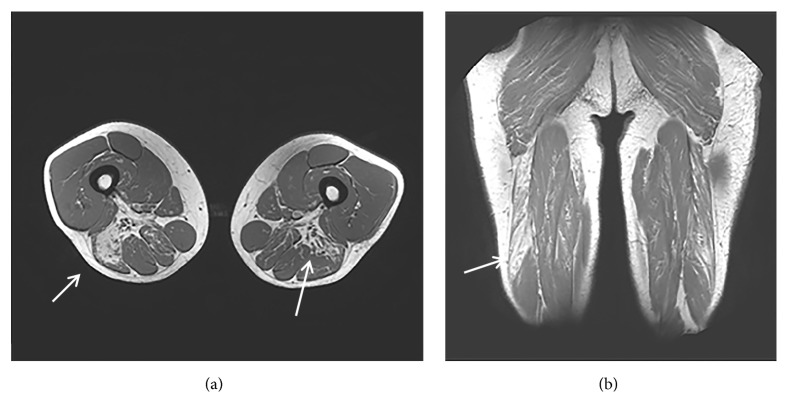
MRI of the thighs in axial T2 (a) and coronal T2 (b) sections shows bilateral fibroadipose degeneration of the* adductor* and* biceps femoris* muscles.
